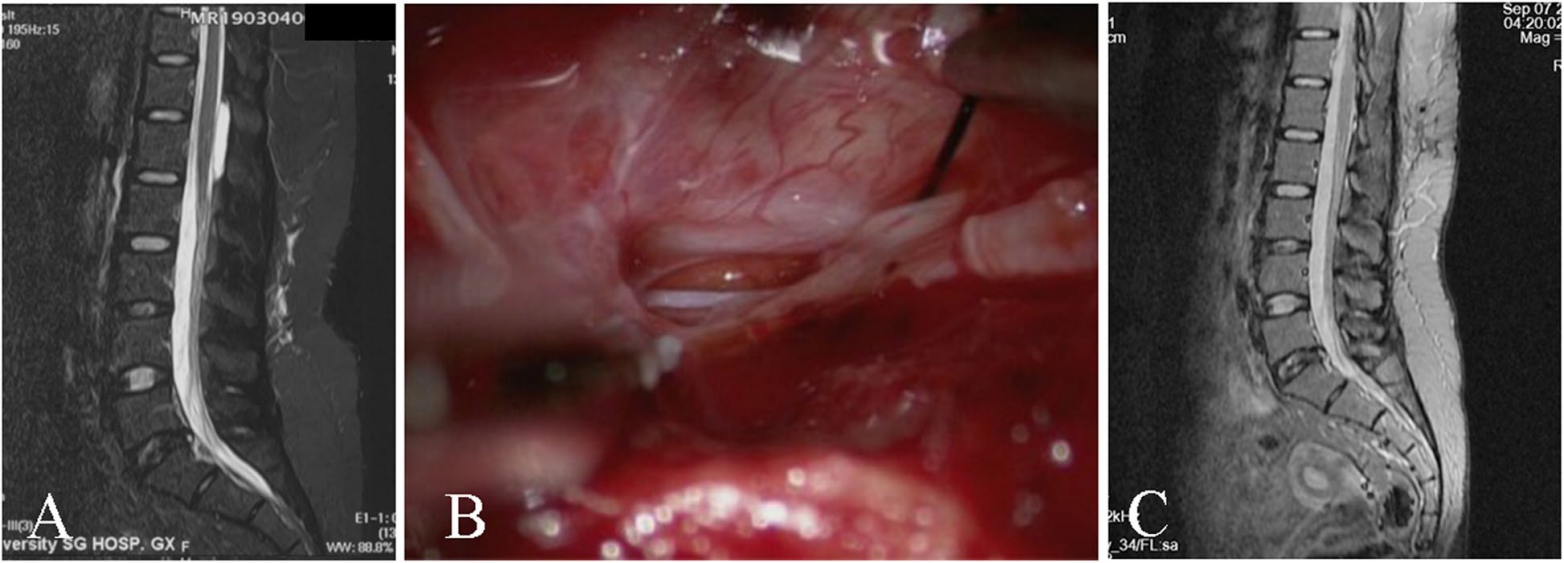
A 26-year-old woman with lower back and left buttock pain for 10 years, aggravating for 1 year. Preoperative MRI showed a SEMC. The dural defect was sutured intraoperatively. One-year follow-up imaging showed no recurrence of the cyst
